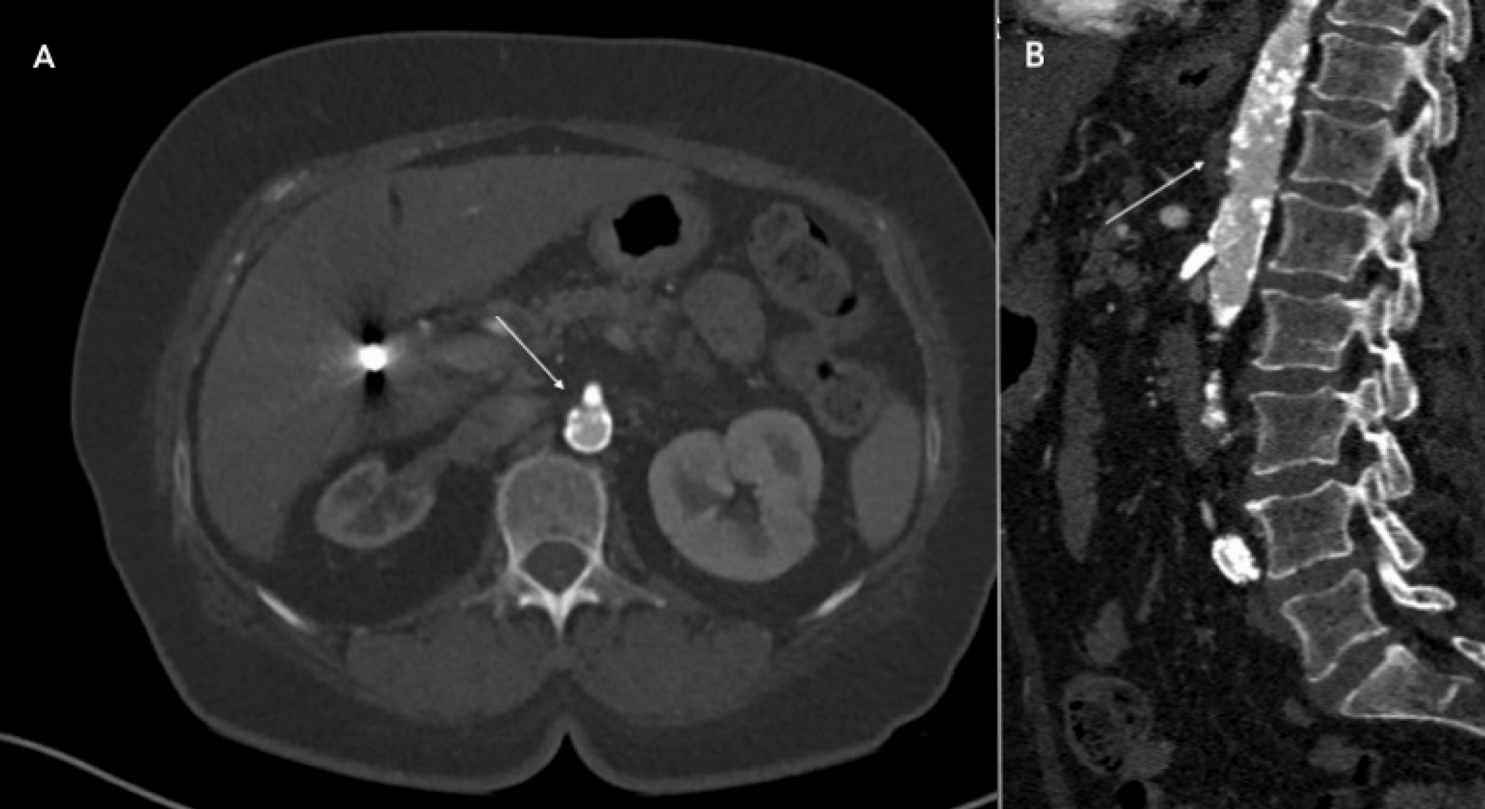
Superior mesenteric artery (SMA) occlusion on computed tomography angiogram (CTA). (A) Axial image showing circumferential calcification of the aorta (white arrow) with occlusive plaque at the ostium of the SMA. (B) Sagittal view of the aorta and SMA origin, demonstrating diffuse luminal calcifications of the aorta (white arrow) and a long occlusion of the origin and proximal SMA.
